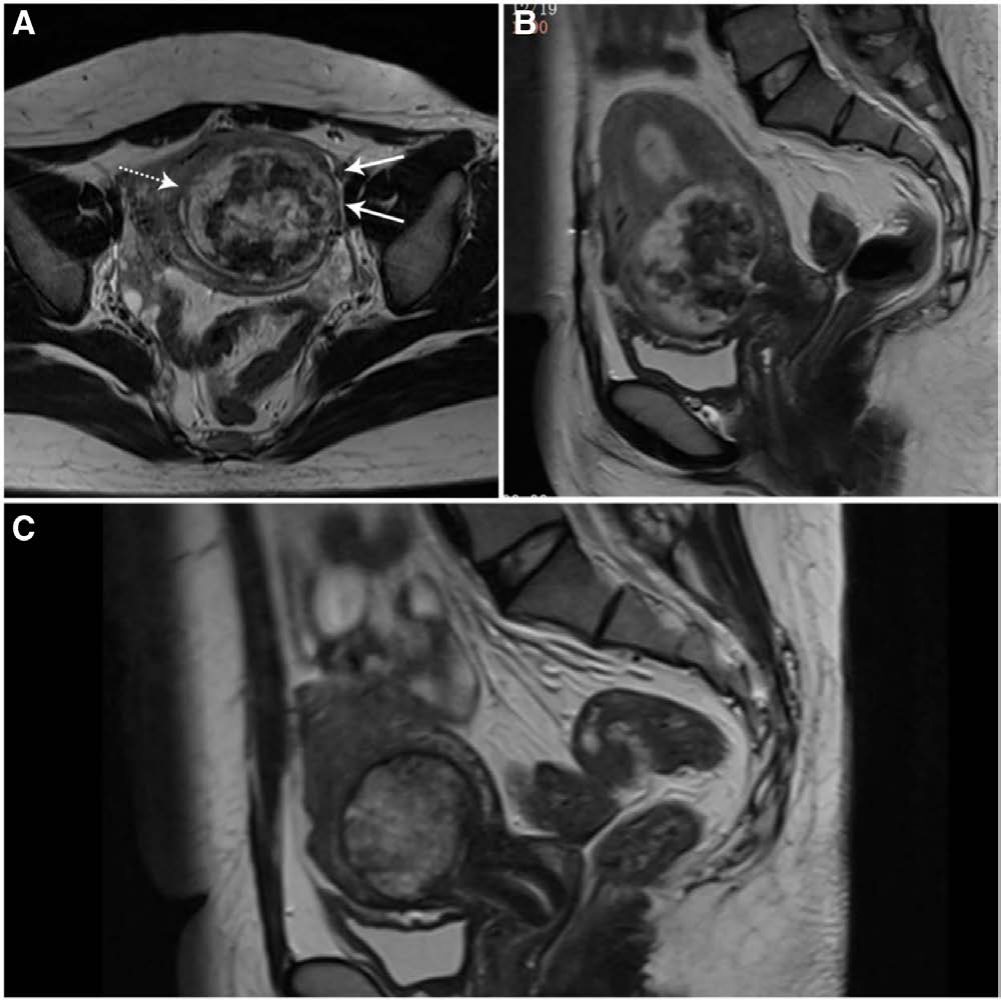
MRI images for the choriocarcinoma patient. (A) Coronal T2-weighted MRI showing the mass at the C-section scar with abundant blood flow signals around the lesion (solid arrow) and well-defined tumor margins (dashed arrow). (B) Sagittal T2-weighted MRI showed a mass without intratumoral vascularity due to severe central necrosis and hemorrhage. (C) Sagittal T2-weighted MRI images showed a gradual reduction of the solid uterine mass after 2 months of chemotherapy. MRI = magnetic resonance imaging.
